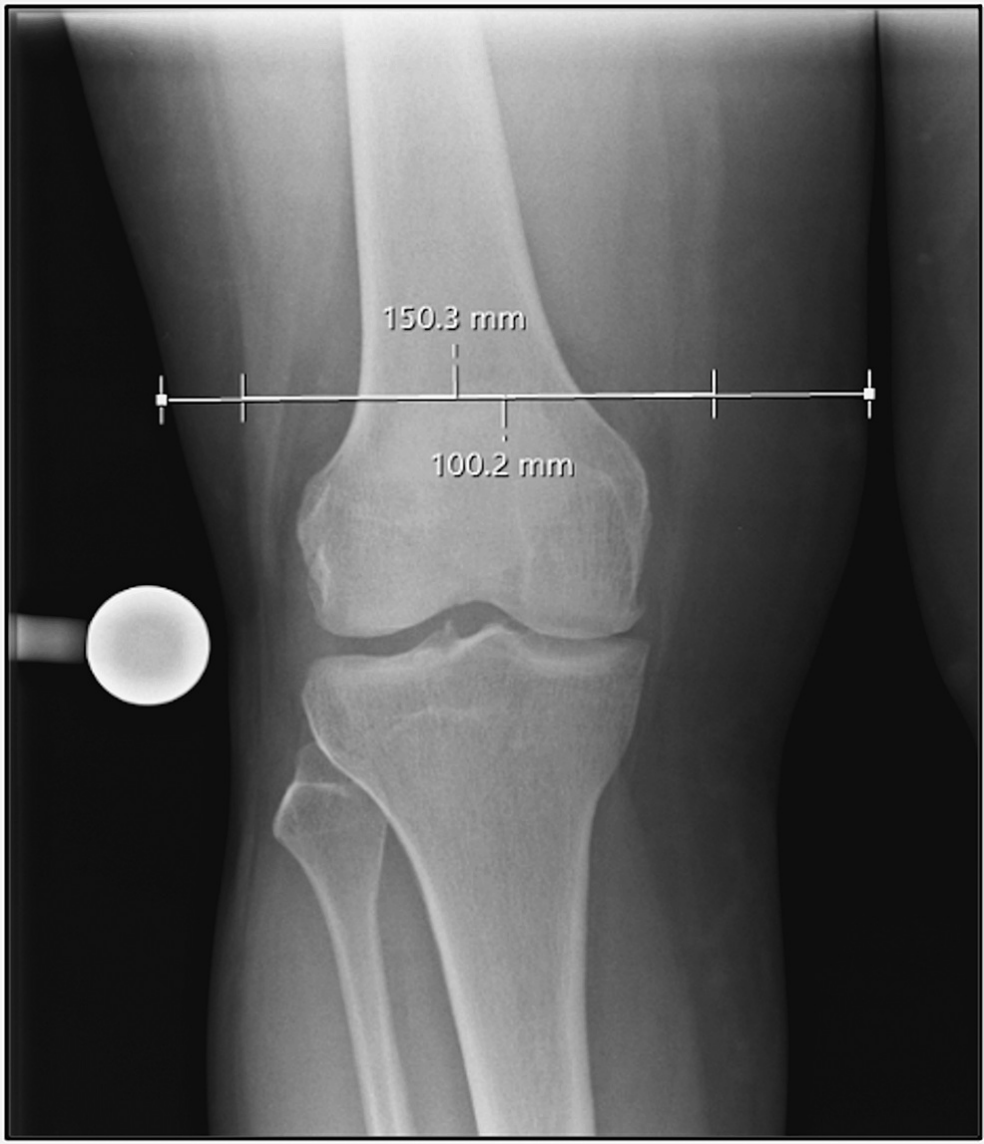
Suprapatellar fat index measurement on weight-bearing anteroposterior knee radiograph.
